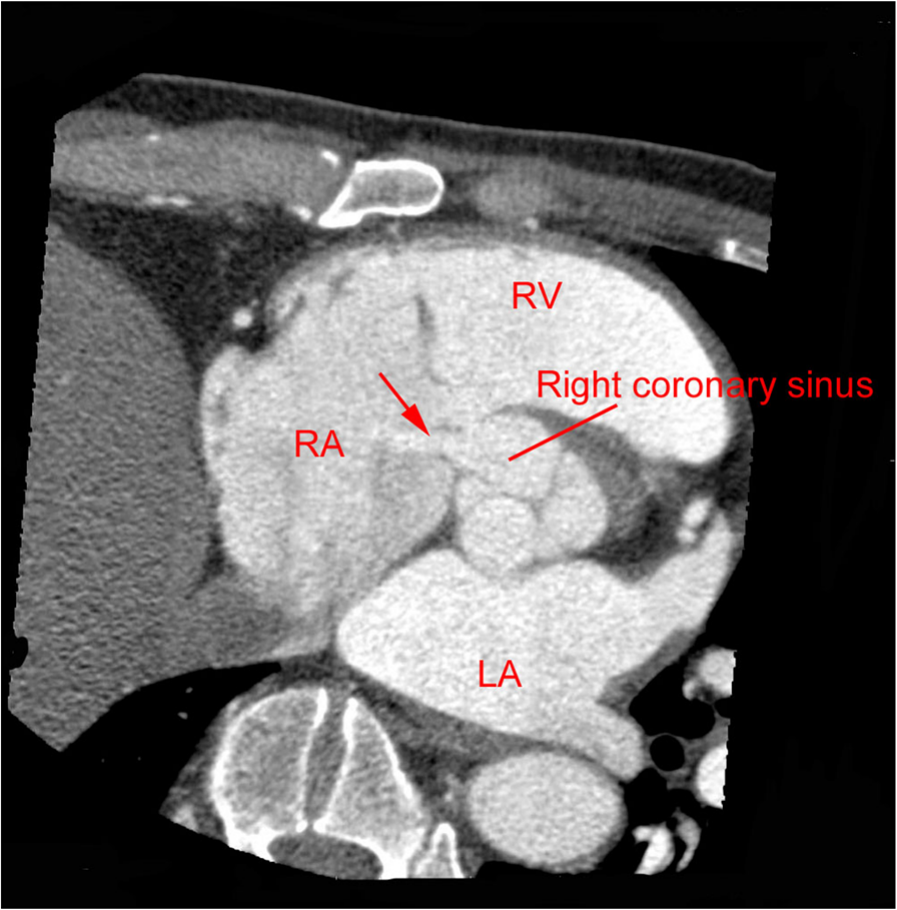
Preoperative computed tomography showing a defect between the right coronary sinus and the right atrium (*arrow*). *LA* left atrium, *RA* right atrium, *RV* right ventricle
